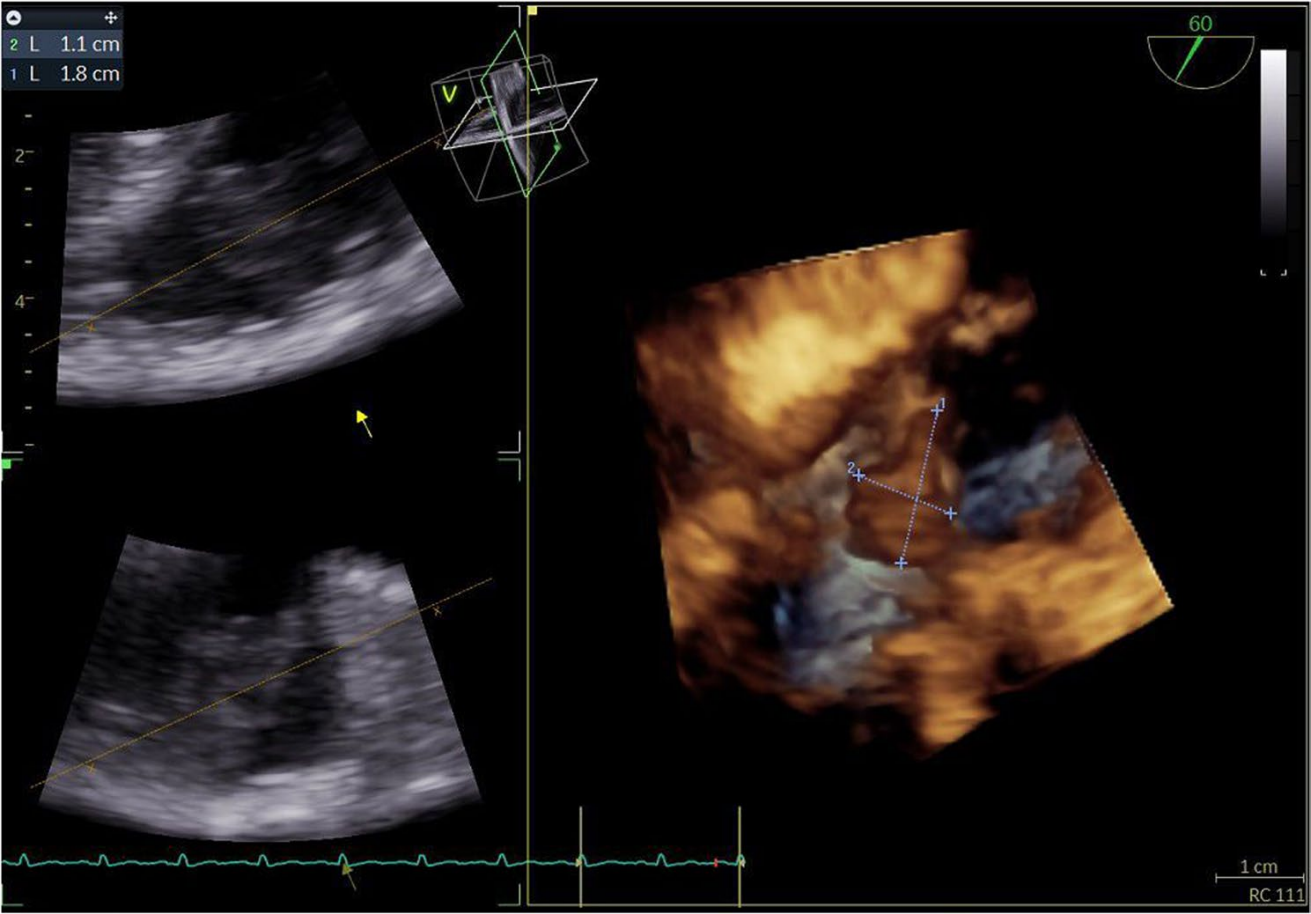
Transesophageal echocardiogram. 60º. 3D reconstruction of the vegetation on the pulmonary valve
